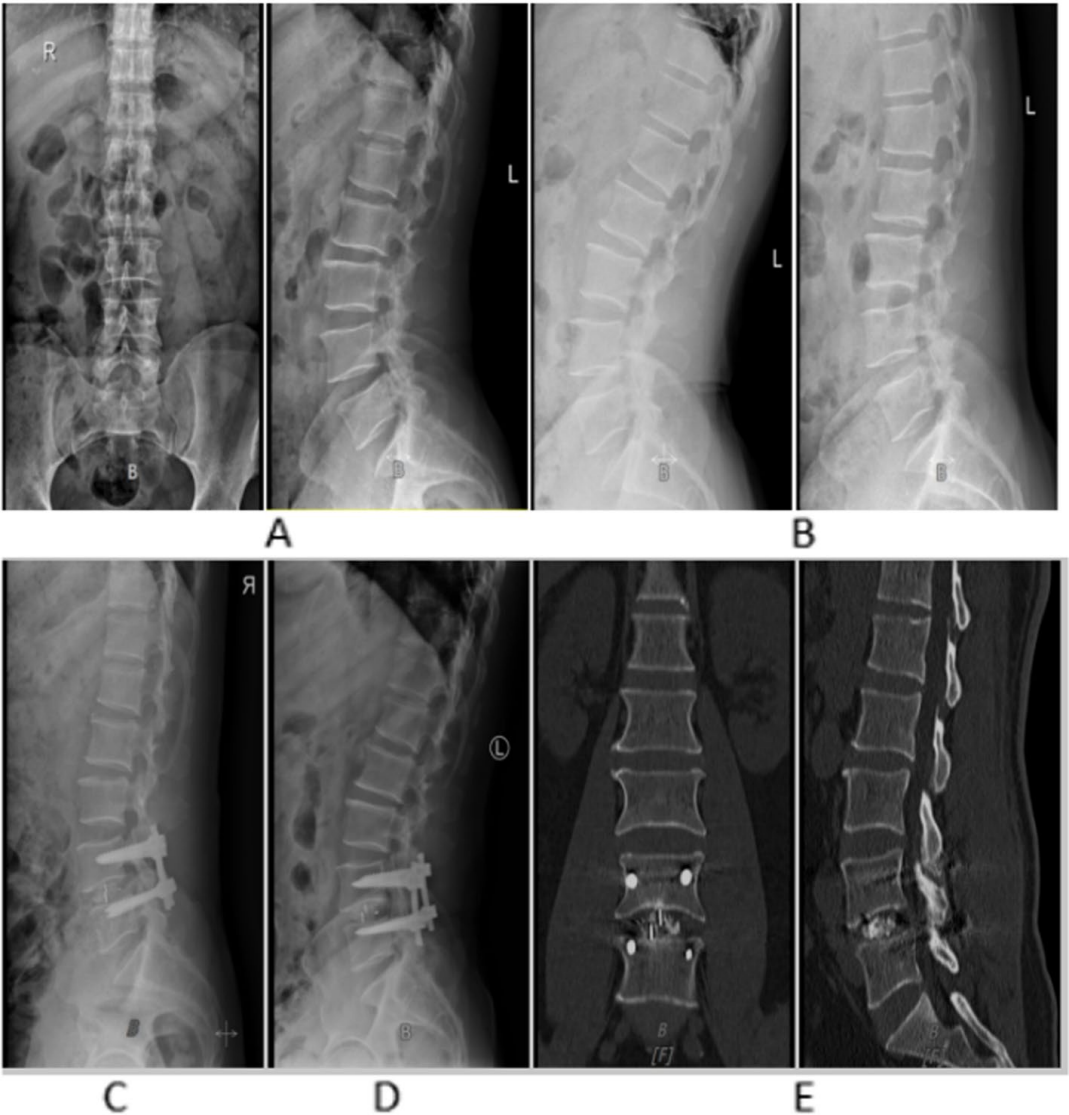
A 56-year-old nonosteoporotic man with lumbar spinal stenosis (forearm T-score = − 1.5) underwent PLIF. A Preoperative anterior–posterior and lateral lumbar X-rays. B Preoperative flexion and extension lumbar X-rays. C Lateral lumbar X-ray at 1 week postoperatively. D and E No subsidence of the cage was observed in X-ray and CT images at 1 year postoperatively
